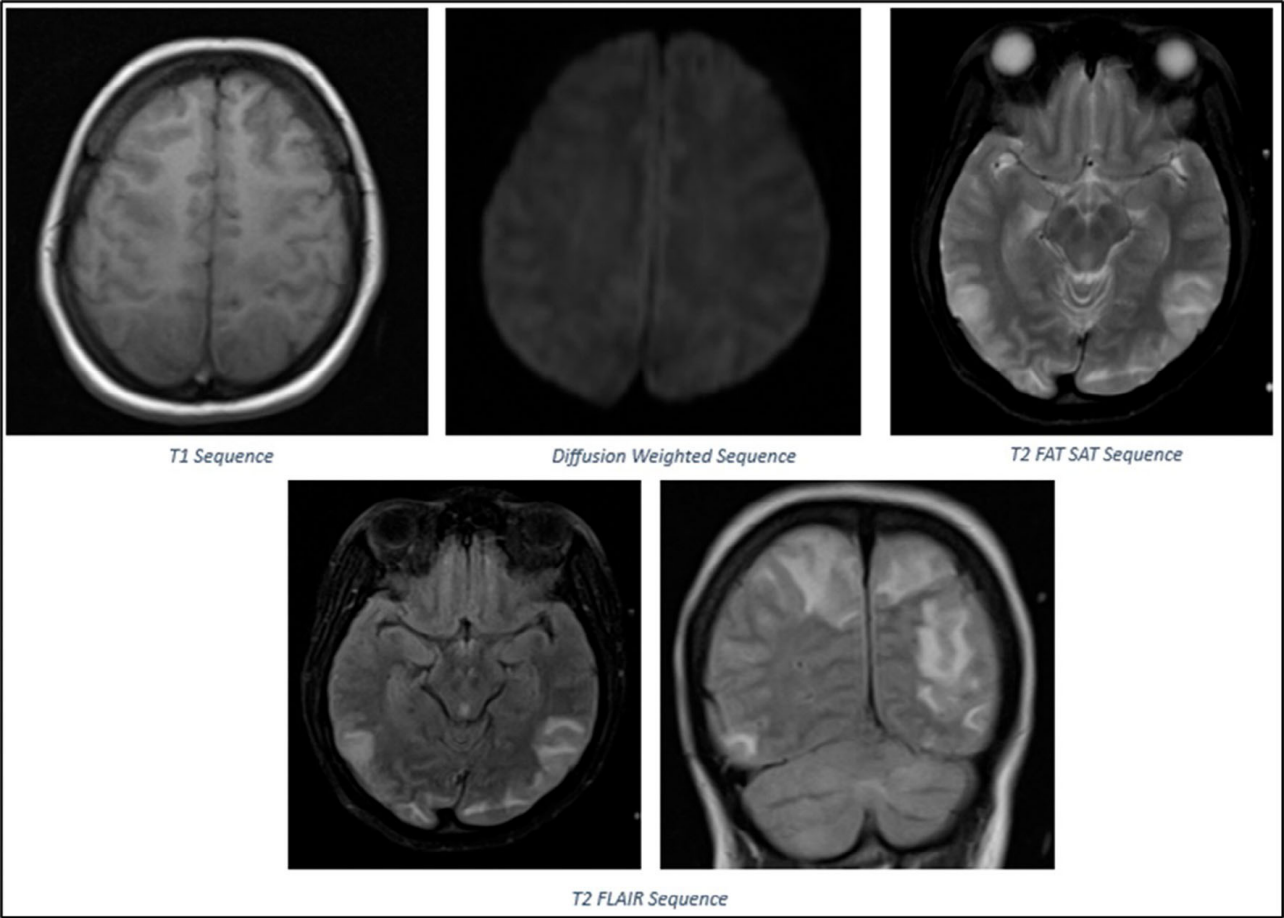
MRI Brain Findings: Cortical and Subcortical T2/FLAIR hyperintensities with oedema in bilateral high frontal, posterior parietal, occipital regions, and bilateral cerebellar hemispheres consistent with PRES.
